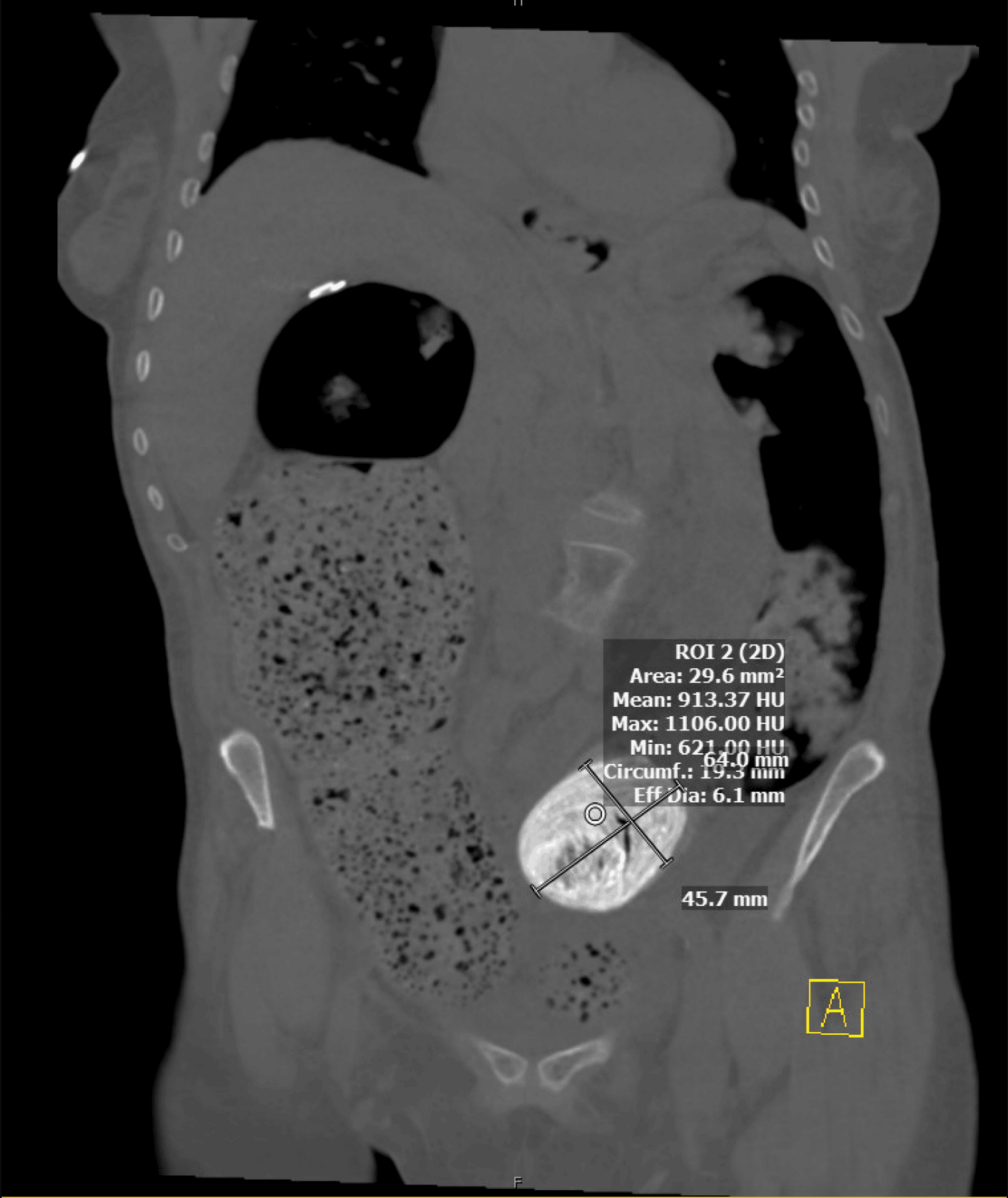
CT imaging in the coronal plane revealing a large barolith lodged in the sigmoid colon with upstream dilation of the cecum and transverse colon, and the distal sigmoid is collapsed
